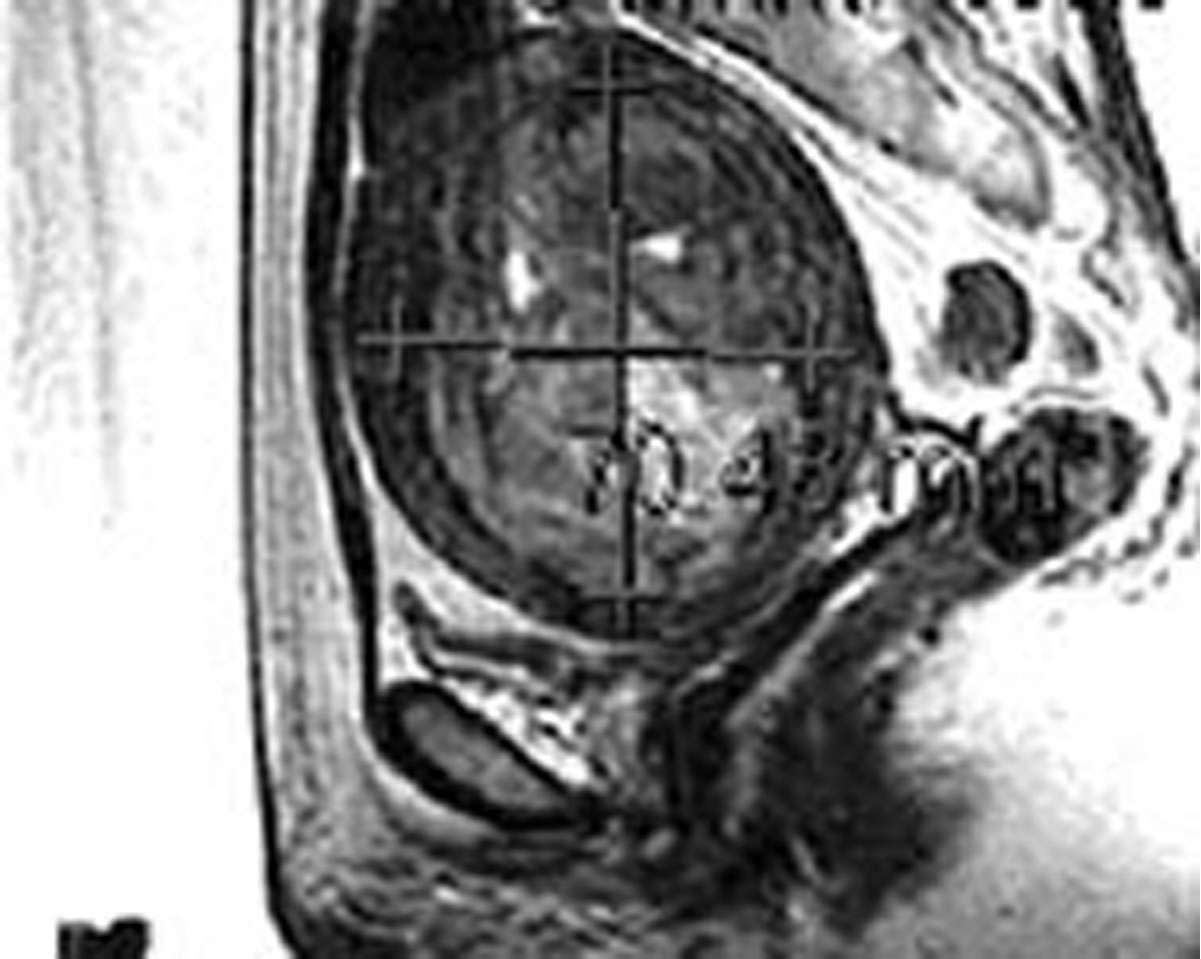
Sagittal T2-weighted image following 3 months of GnRH agonist therapy, showing a 281 cc fibroid representing a 40% reduction in volume.
